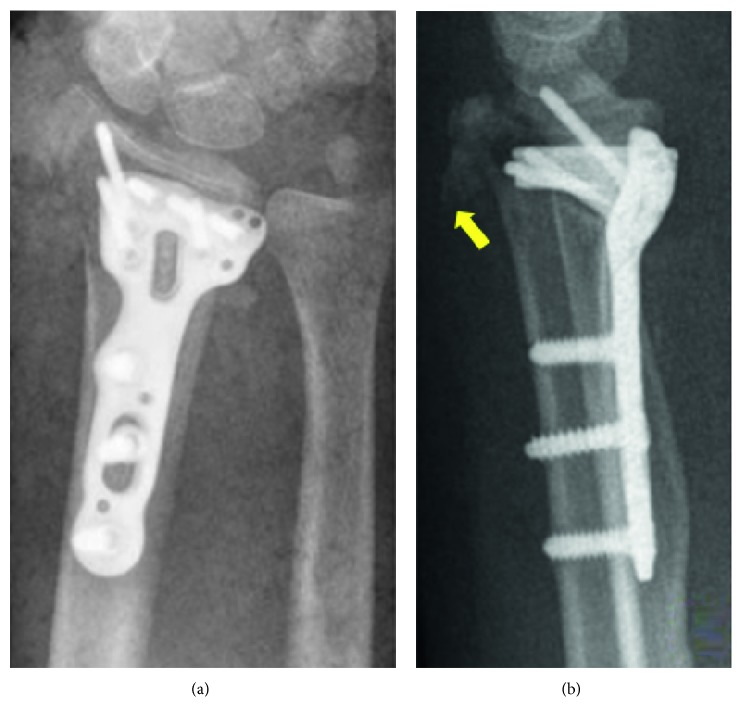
Immediate postoperative X-rays showing internal fixation (a). Lateral view showing dorsal metaphyseal comminution (arrow) (b).
